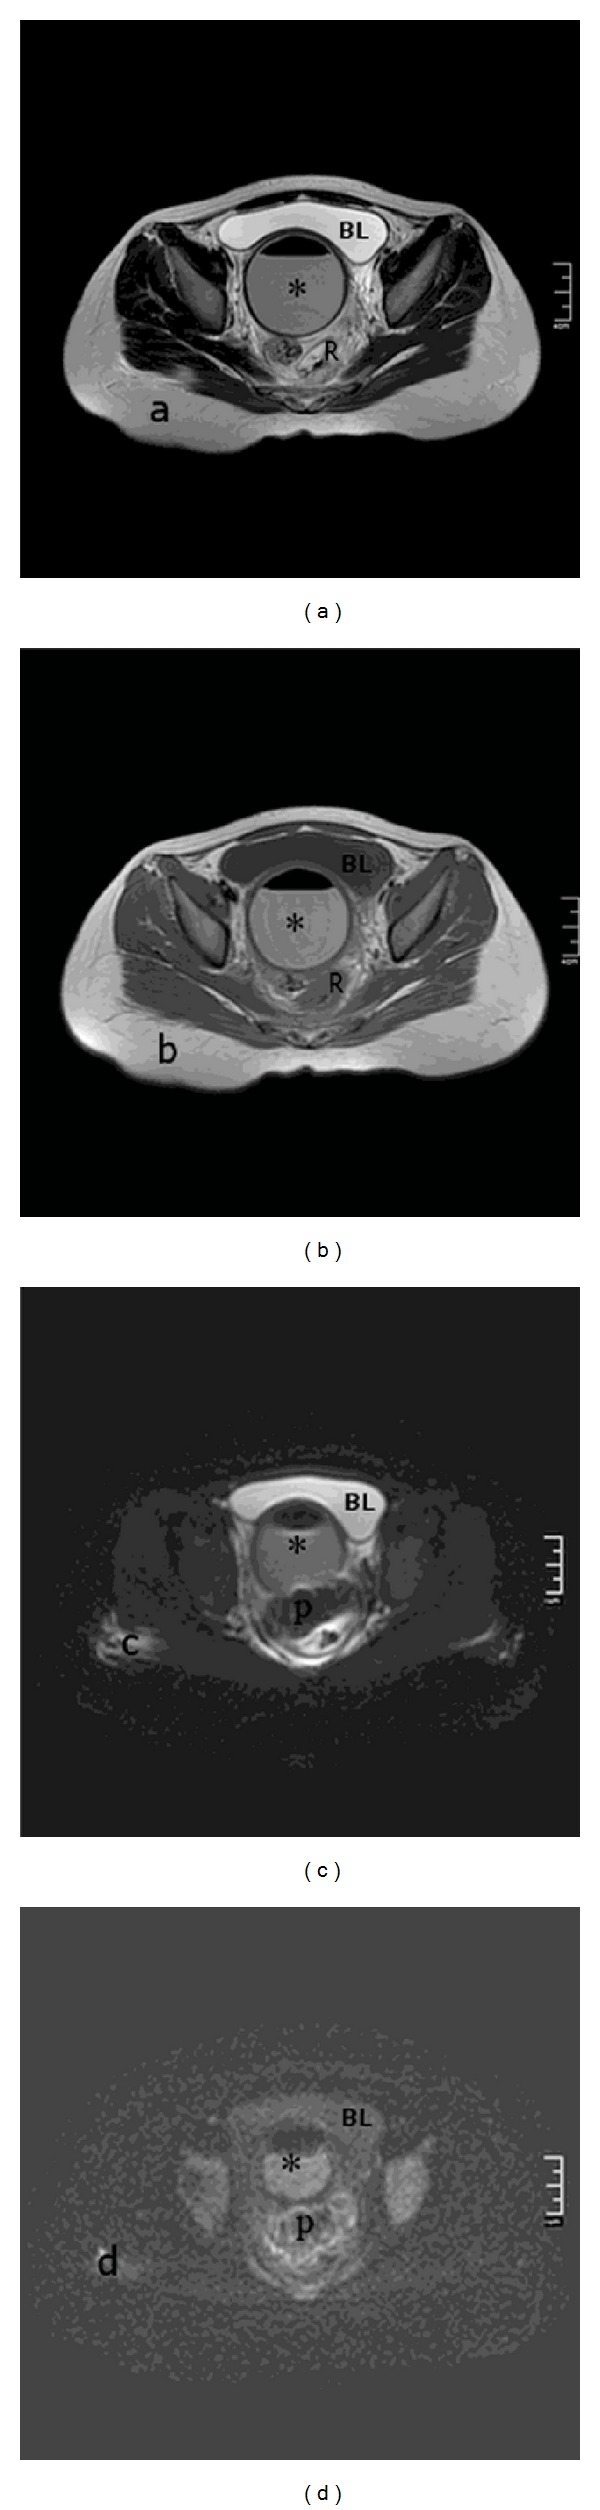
(a) Axial T2-weighted FSE image. Material with air-fluid level shows lower signal-intensity than bladder (BL). (b) Axial T1-weighted FSE image. Material with air-fluid level shows higher signal-intensity than bladder (BL), ((c), (d)) apparent diffusion coefficient (ADC), and diffusion-weighted image (DWI). Restricted diffusion indicates purulent collection in the rectovaginal space, pus (P), and vagina (*) rectum was compressed by the rectovaginal space leakage posterolaterally, rectum (R).
